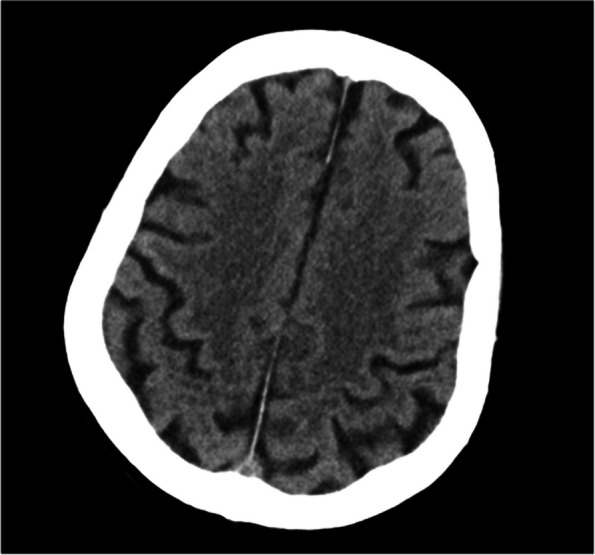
Head computed tomography scan showing bilateral lacunar cerebral infarction in the basal ganglia
